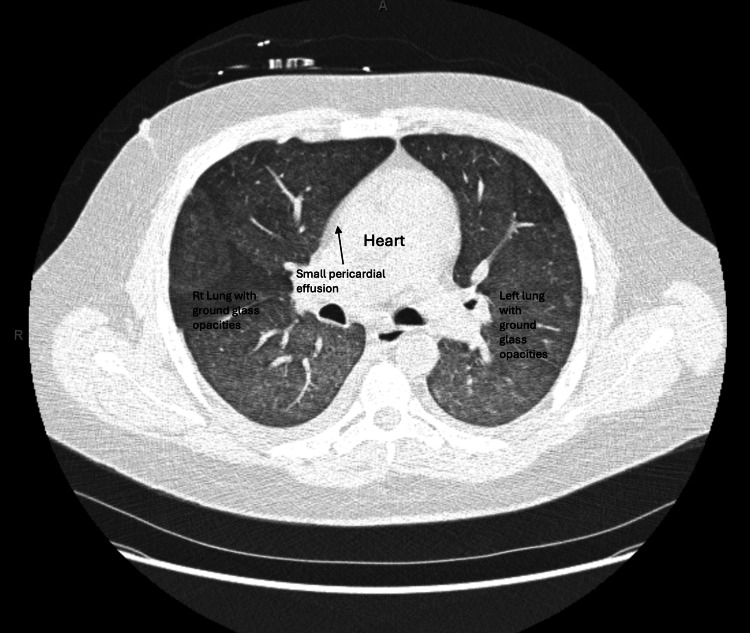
Axial view of CT scan of the chest. The image shows trace pericardial effusion and bilateral ground-glass lung opacities.
